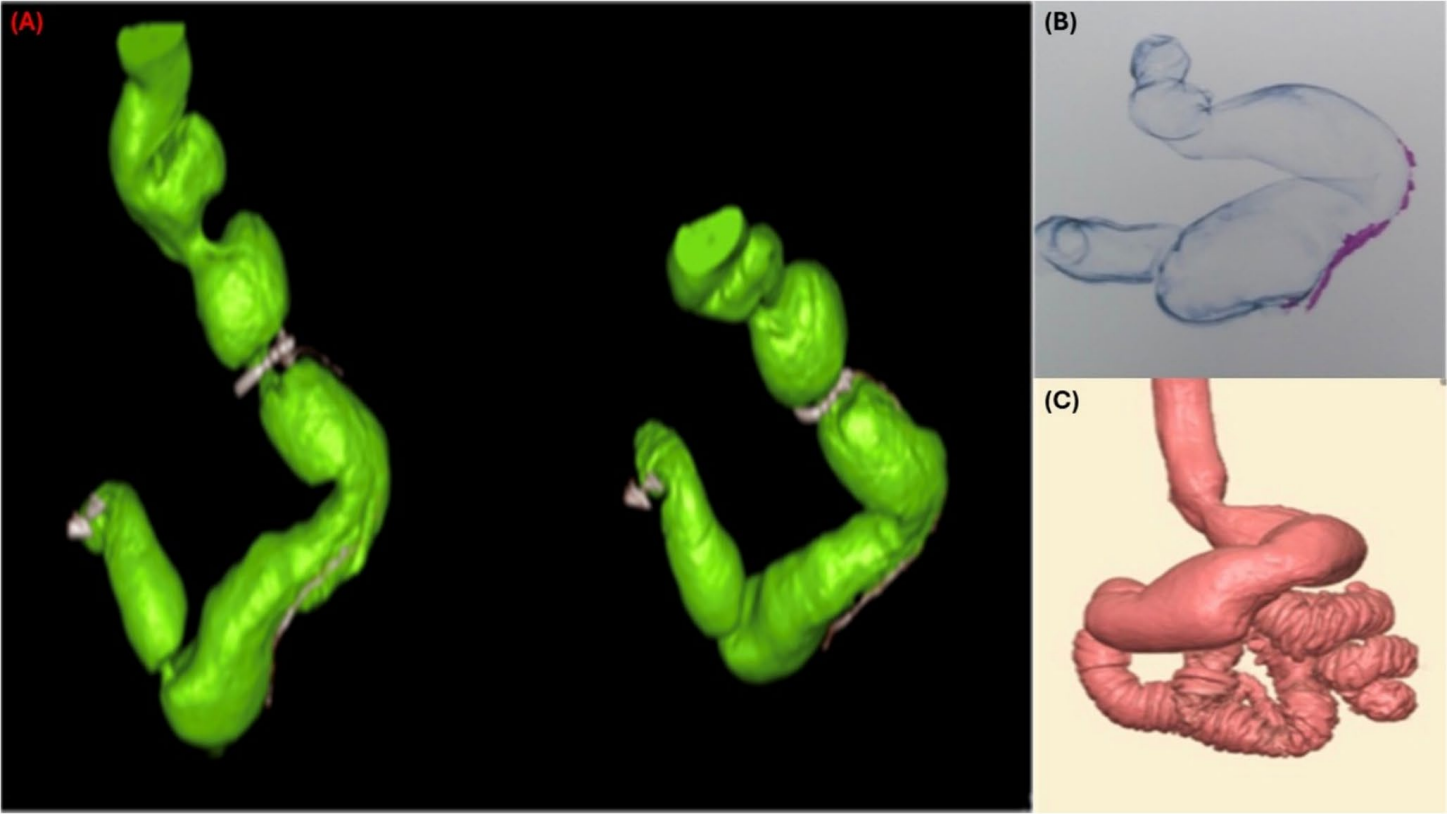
Postoperative 3-D CT volumetry of the gastric sleeve at 36-month follow-up. Surface-rendered reconstructions after oral contrast with measured pouch volumes: (A) ring-augmented sleeve gastrectomy (Ra-SG), 120 mL (two views of the same case; ring visible; small sliding hiatal hernia), (B) standard sleeve gastrectomy (SG), 160 mL, (C) SG, 180 mL
